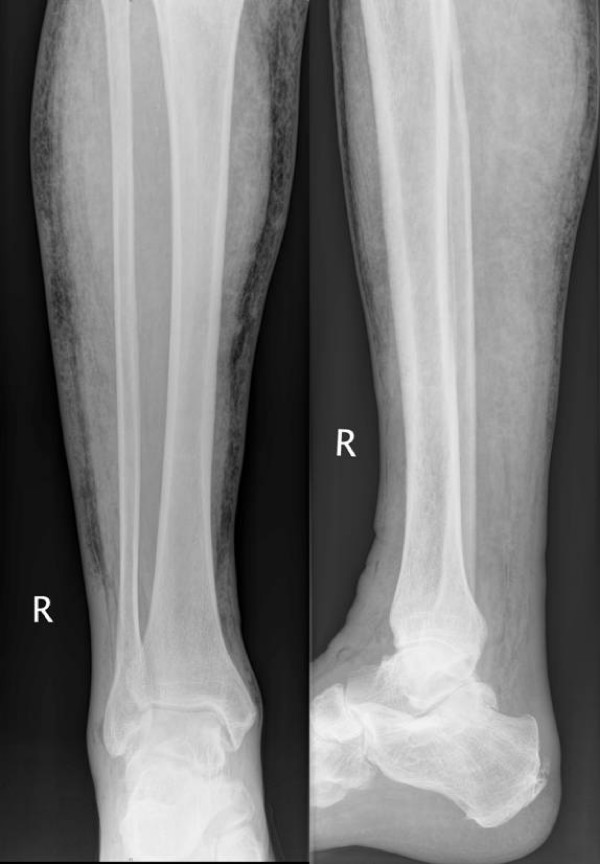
X-Ray Images of the right lower leg of patient three. X-Ray Images in the anteroposterior and lateral projection of the right lower leg and ankle joint of the third patient: Free gas in the subcutaneous and in the muscle tissue can be seen. According to these images, the surgeon in charge of another hospital admitted the patient to our department with the provisional diagnosis "gas gangrene of the right leg" for amputation and intensive care monitoring.
